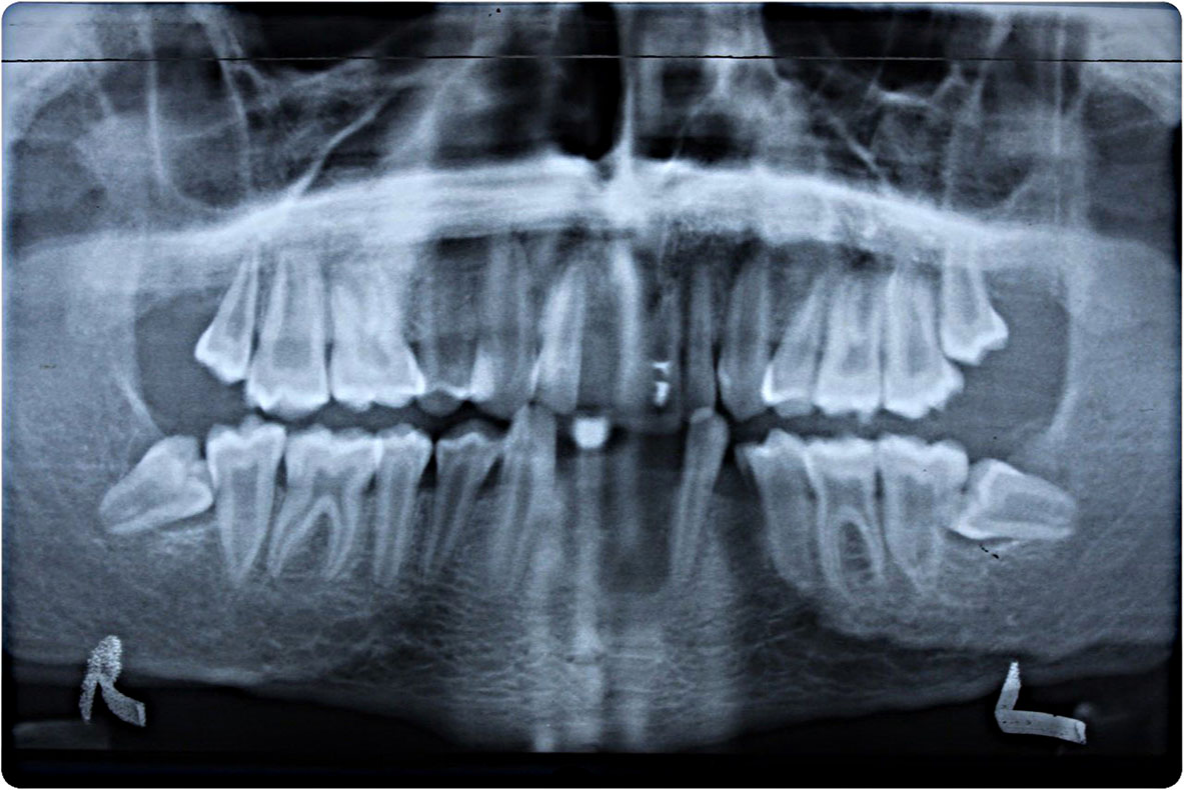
Pre-operative radiographs. Radiographic situation at presentation
